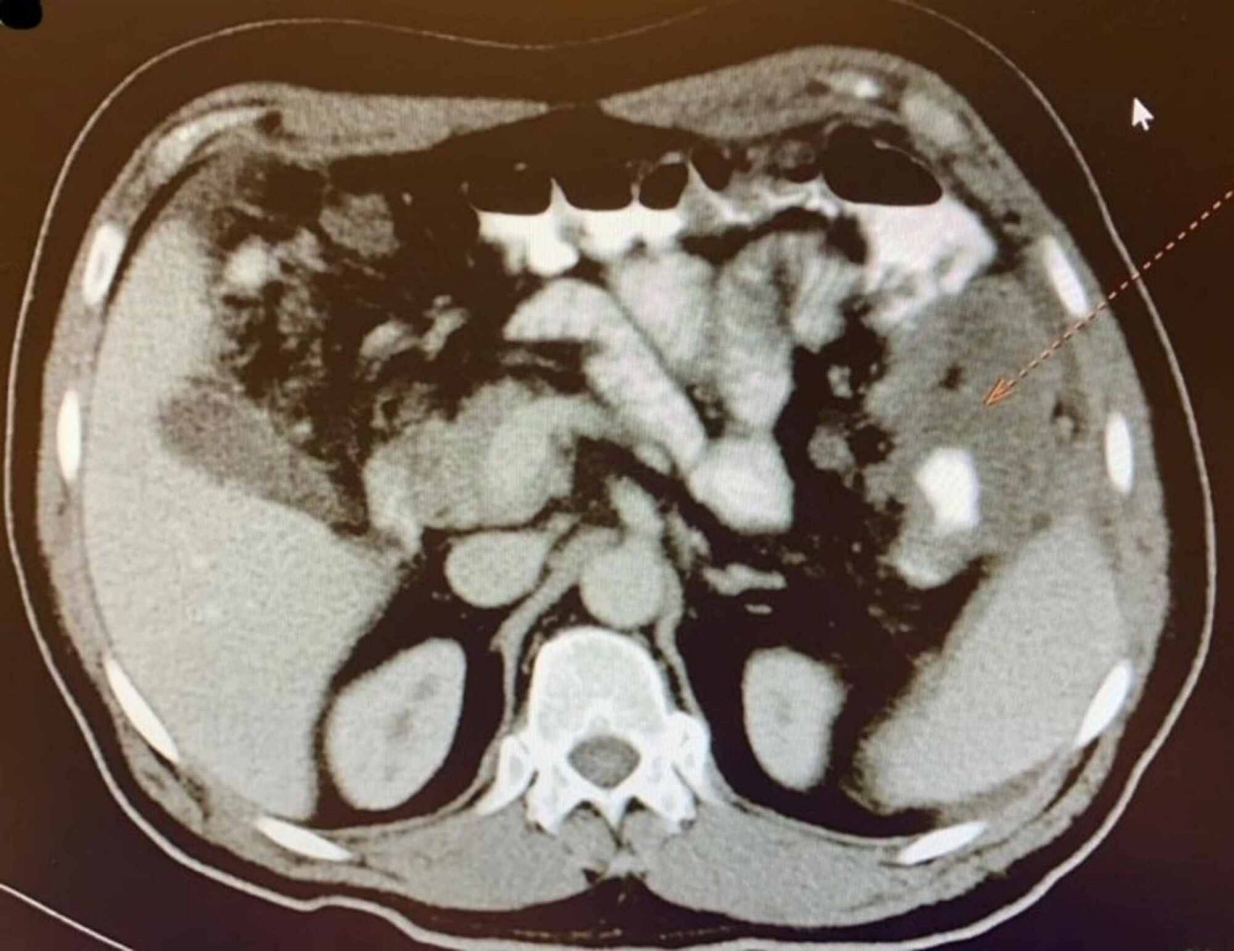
A cross-sectional slice of CT abdomen showing left colonic mass with extramural extension.
